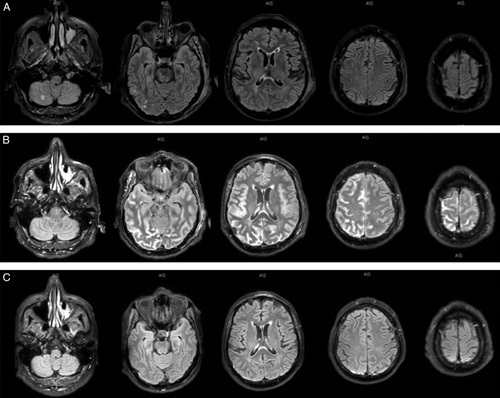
Fluid attenuated inversion recovery scans. (A) Magnetic resonance imaging (MRI) performed on day 1 showed punctate lesions in the cerebellum bilaterally and a small right parieto-temporal hematoma. (B) MRI performed on day 2 showed (in addition to the parietal hematoma) diffuse hyperintensity of the sulcal regions of the right hemisphere, left posterior parietal and fronto-mesial regions, and a linear right pachymeningeal enhancement (C) magnetic resonance performed on day 14 showed a slight right posterior pachimeningeal enhancement.
